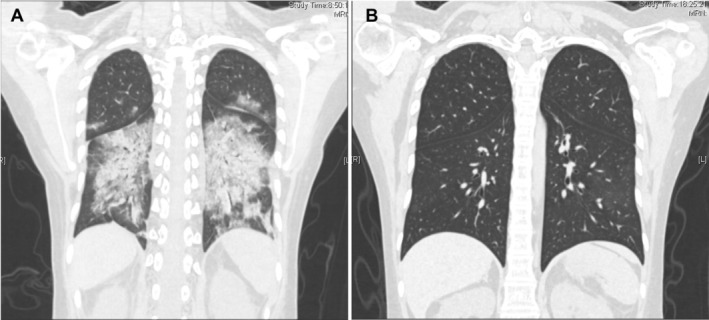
(A) Chest computed tomography (CT) scan obtained on admission showing multiple patchy and streaky areas of increased attenuation in both lungs, accompanied by bilateral ground‐glass opacities. (B) Follow‐up chest CT scan at 3 months demonstrating complete radiological resolution of the pulmonary lesions.
